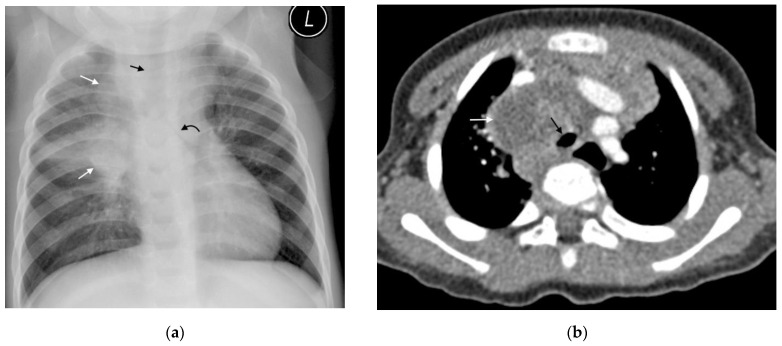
(a,b): Lymphadenopathy, air-space disease and airway compression on chest radiograph and CT. (a) Frontal AP chest radiograph in a 14-month-old boy with confirmed pulmonary TB demonstrating right hilar and paratracheal lymphadenopathy as lobulated masses projecting from the right of the cardio-mediastinal shadow (white arrows). There is also air-space disease in the right upper lobe, tracheal compression (black arrow) and left main bronchus compression (curved black arrow), resulting from presumed subcarinal and left hilar lymphadenopathy. (b) Axial post-contrast CT scan confirming the right paratracheal lymphadenopathy which has a low-density centre and fine rim enhancement (white arrow) and AP compression of the trachea (black arrow), which was not appreciated on the AP radiograph.
